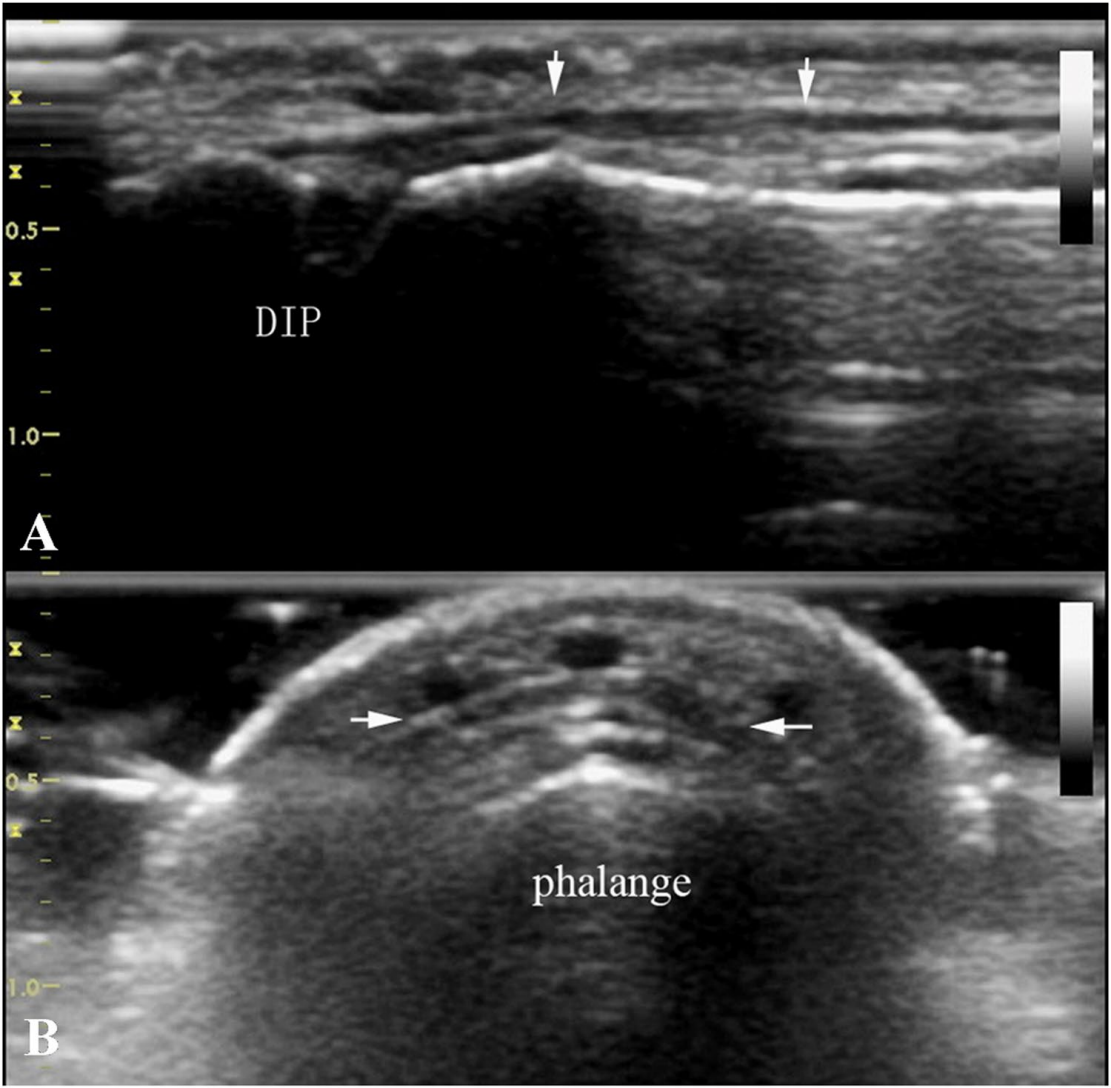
Normal extensor tendon of finger. High frequency ultrasonography image of extensor tendon in longitudinal plane (Fig. 1A) and transverse plane (Fig. 1B).
